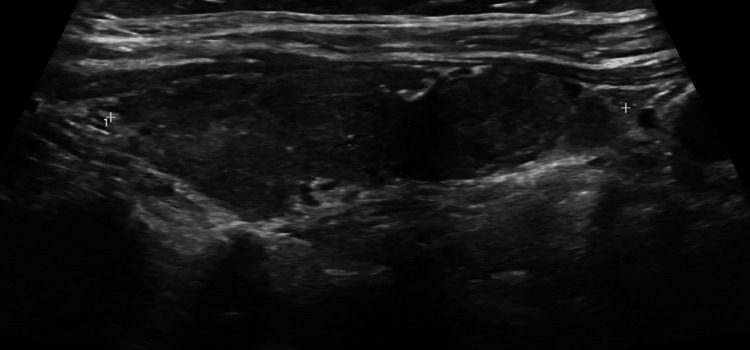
Thyroid ultrasound findings Sagittal ultrasound image of the left thyroid lobe demonstrated no dominant or discrete nodules.
